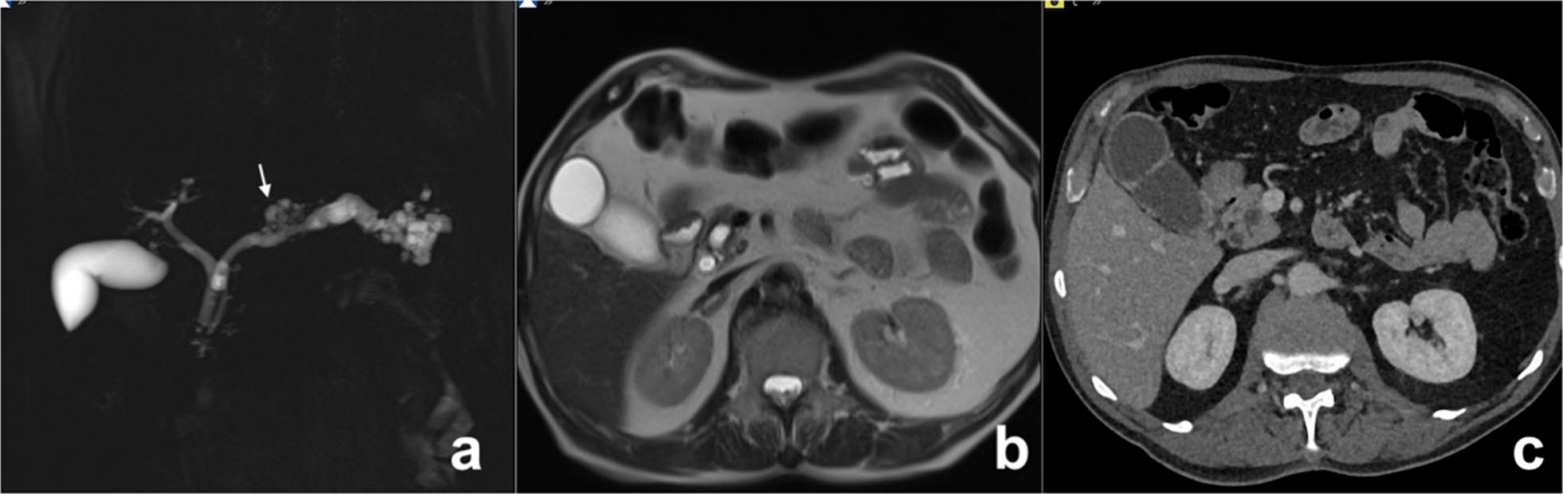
Diffuse MD-IPMN. At MRCP (a), a diffuse enlargement of the MPD is appreciable through the gland with a grape-like dilatation of branch ducts (arrow) and without significant causes of obstruction nor calcifications both on T2w (b) and CT (c)
